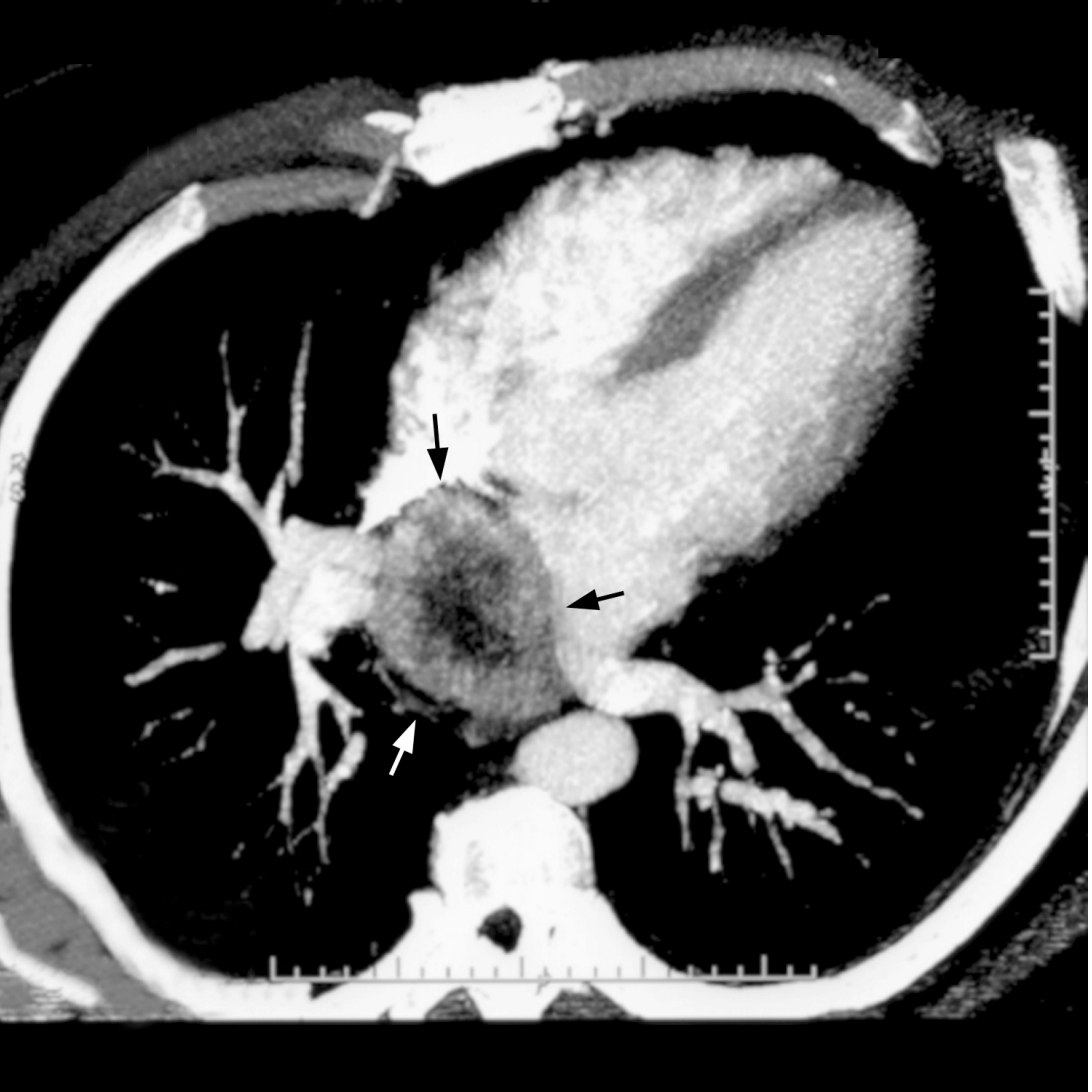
CT scan: image showing a solid and likely necrotic mass (arrows) extending posterior to the left atrium.
